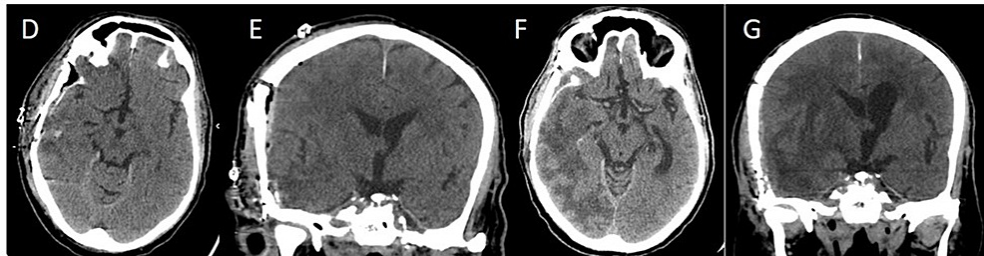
Axial (D) and coronal (E) noncontrast CT images demonstrate post-surgical changes after right-sided craniotomy with evaluation of the hematoma. However, follow-up CT (axial (F) & coronal (G)) nine months later, demonstrate marked right cerebral hemisphere edema centered at right temporal lobe with mass effect on the right lateral ventricle with right to left midline shift.
